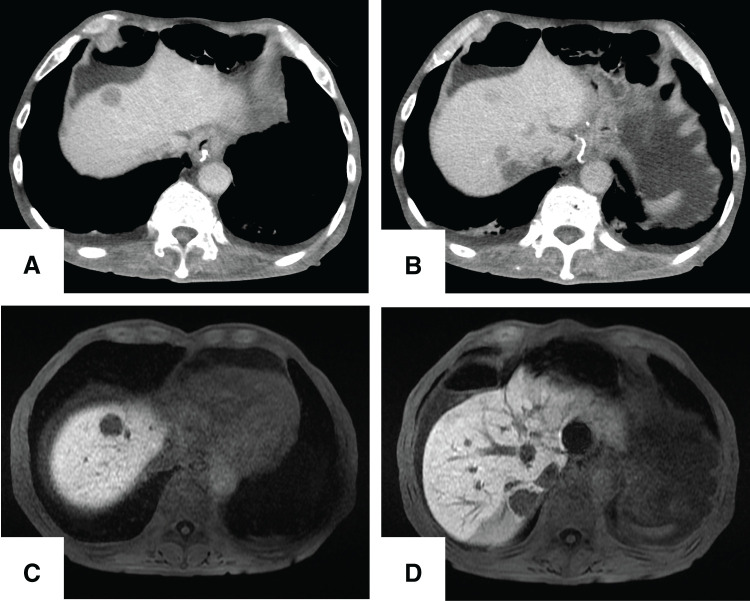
Imaging findings of multiple liver tumors. (A, B) Contrast-enhanced CT showing multiple hypodense liver lesions. (C, D) Ethoxybenzyl (EOB)-MRI (hepatobiliary phase) showing multiple hypointense liver lesions.
